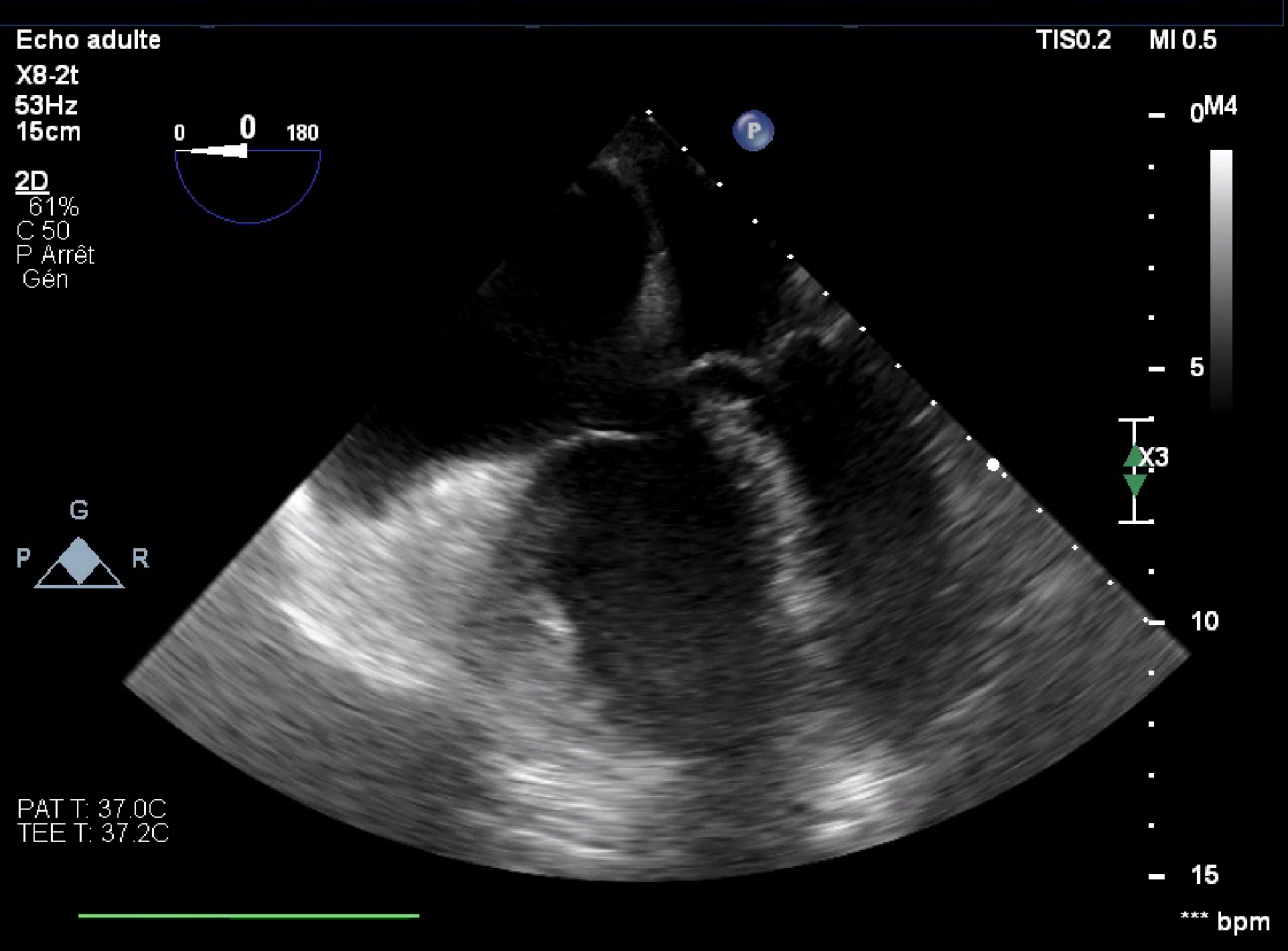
Transesophageal echocardiography after Right ventricle dilatation (ROSC) with hypertrophy of the free wall. Left ventricle is small and hyperdynamic. Pulmonary arterial pressures estimated at 100 mmHg.
